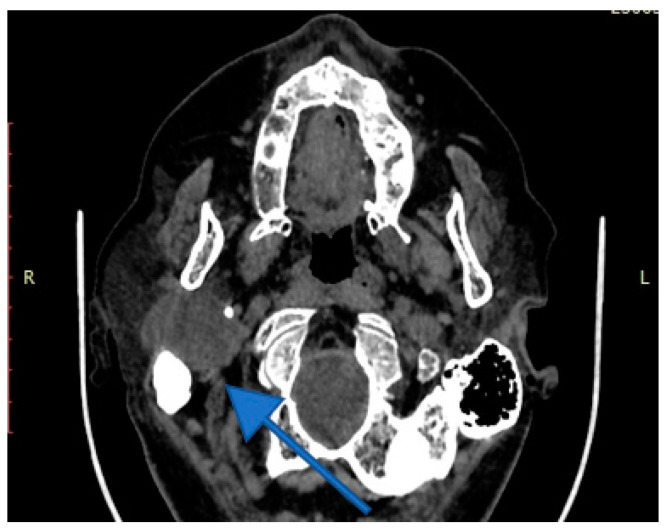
Contrast-enhanced cervical CT view showing deep lobe tumor of the right parotid gland, with extension into the right infratemporal fossa widening the distance between the styloid process and the mastoid process of the temporal bone (arrow).
